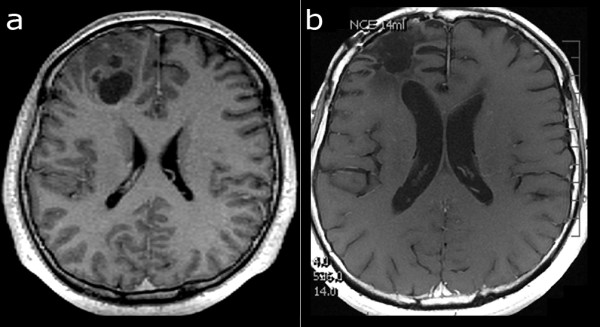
Serial MR imaging in case 3. T1-weighted image after application of contrast agent. a) January 2005 before surgery. GBM located in the right frontal region. b) December 2011. No tumor recurrence detected. GBM, glioblastoma multiforme; MR, magnetic resonance.
